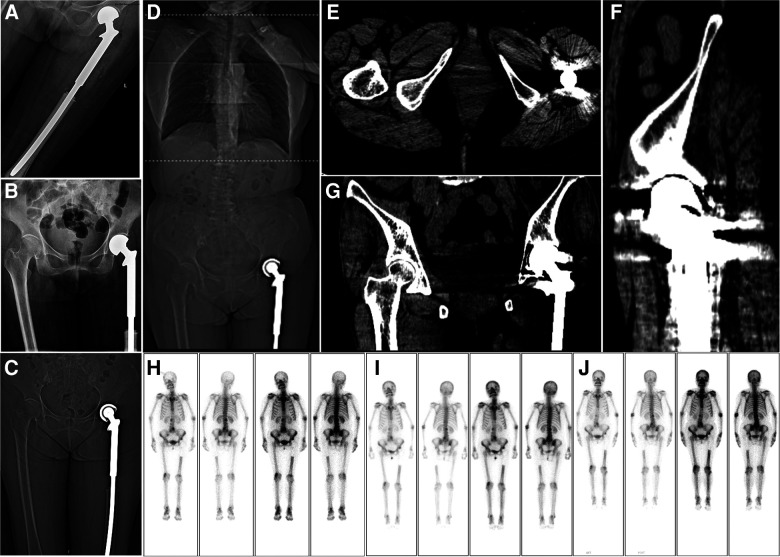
Postoperative imaging. (A,B) A radiograph of the left hip joint shows that the joint prosthesis is in place 1 week after the operation. (C) Coronal view of a computed tomography (CT) scan performed 1 week postoperatively. (D–G) Chest, abdominal, and pelvic CT examinations at 42 months postoperatively show no obvious metastasis in the lungs. Meanwhile, the joint prosthesis is in place, and there is no obvious recurrence in the local area. (H–J) After 13, 24, and 32 months postoperatively, whole-body bone scan findings show no obvious recurrence.
